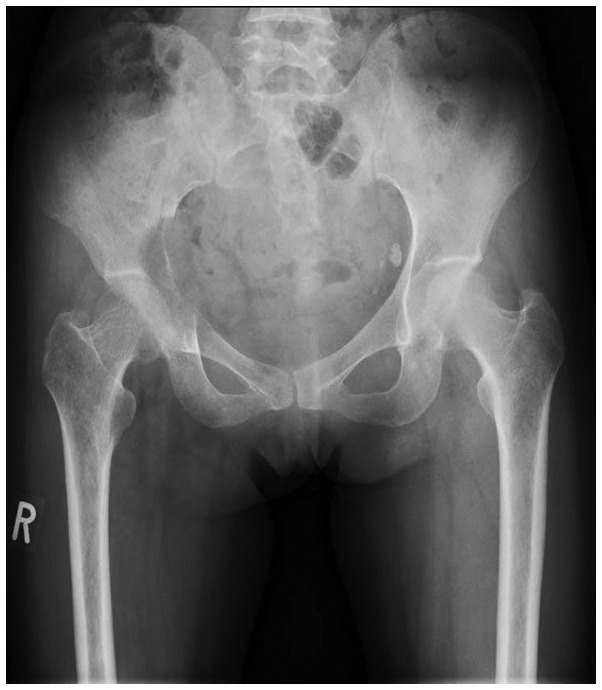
Case one: Plain radiograph showing an osteolytic lesion in the right acetabulum.
